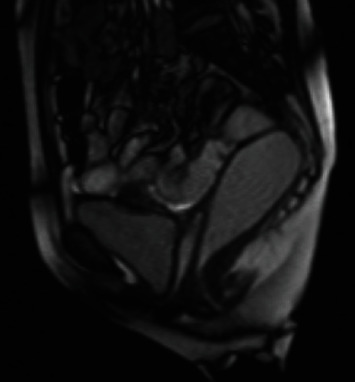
MRI defecography demonstrating anterior compartment prolapse. Anterior compartment descends 1.2 cm below the pubococcygeal line (PCL) with defecation consistent with mild anterior prolapse.
